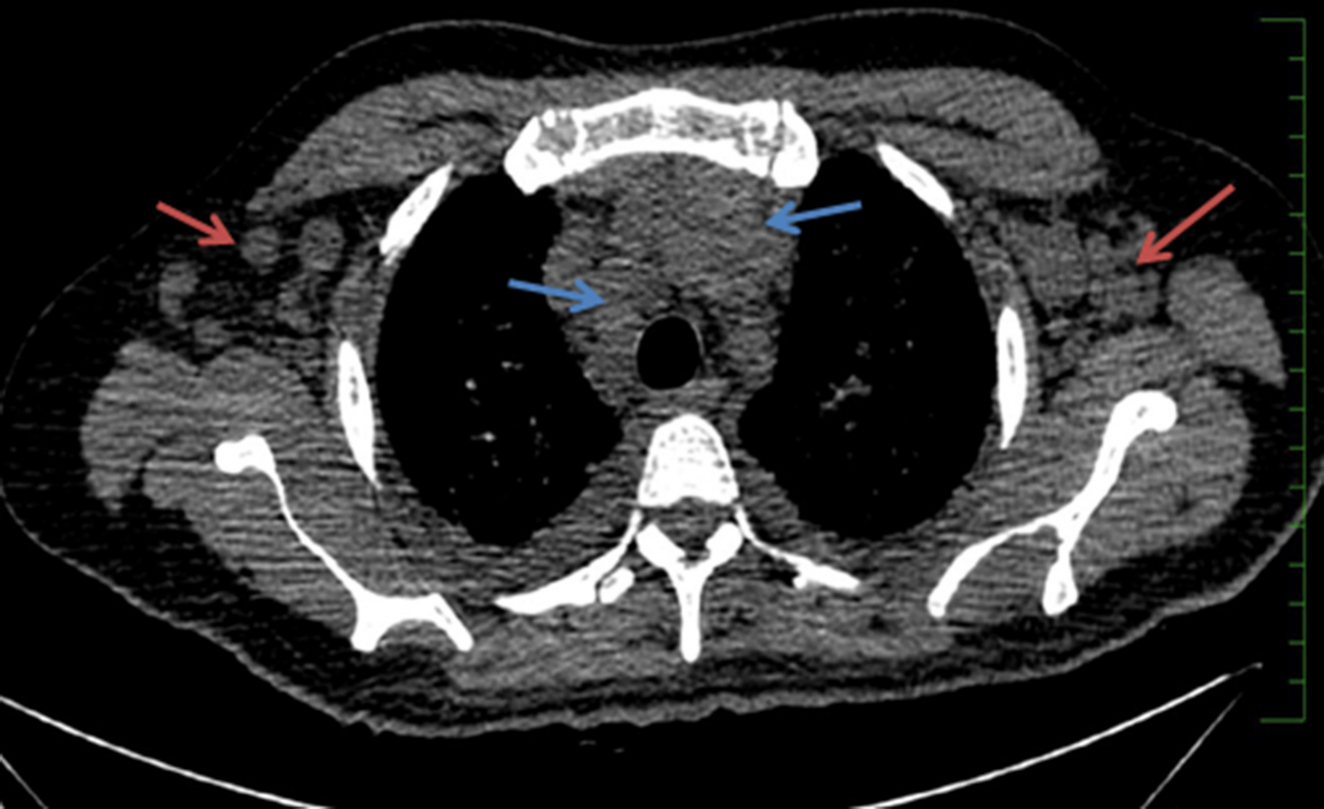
Case 1(c): CT scan of the chest in mediastinal window shows multiple enlarged axillary (red arrows) and mediastinal lymph nodes (blue arrows).
